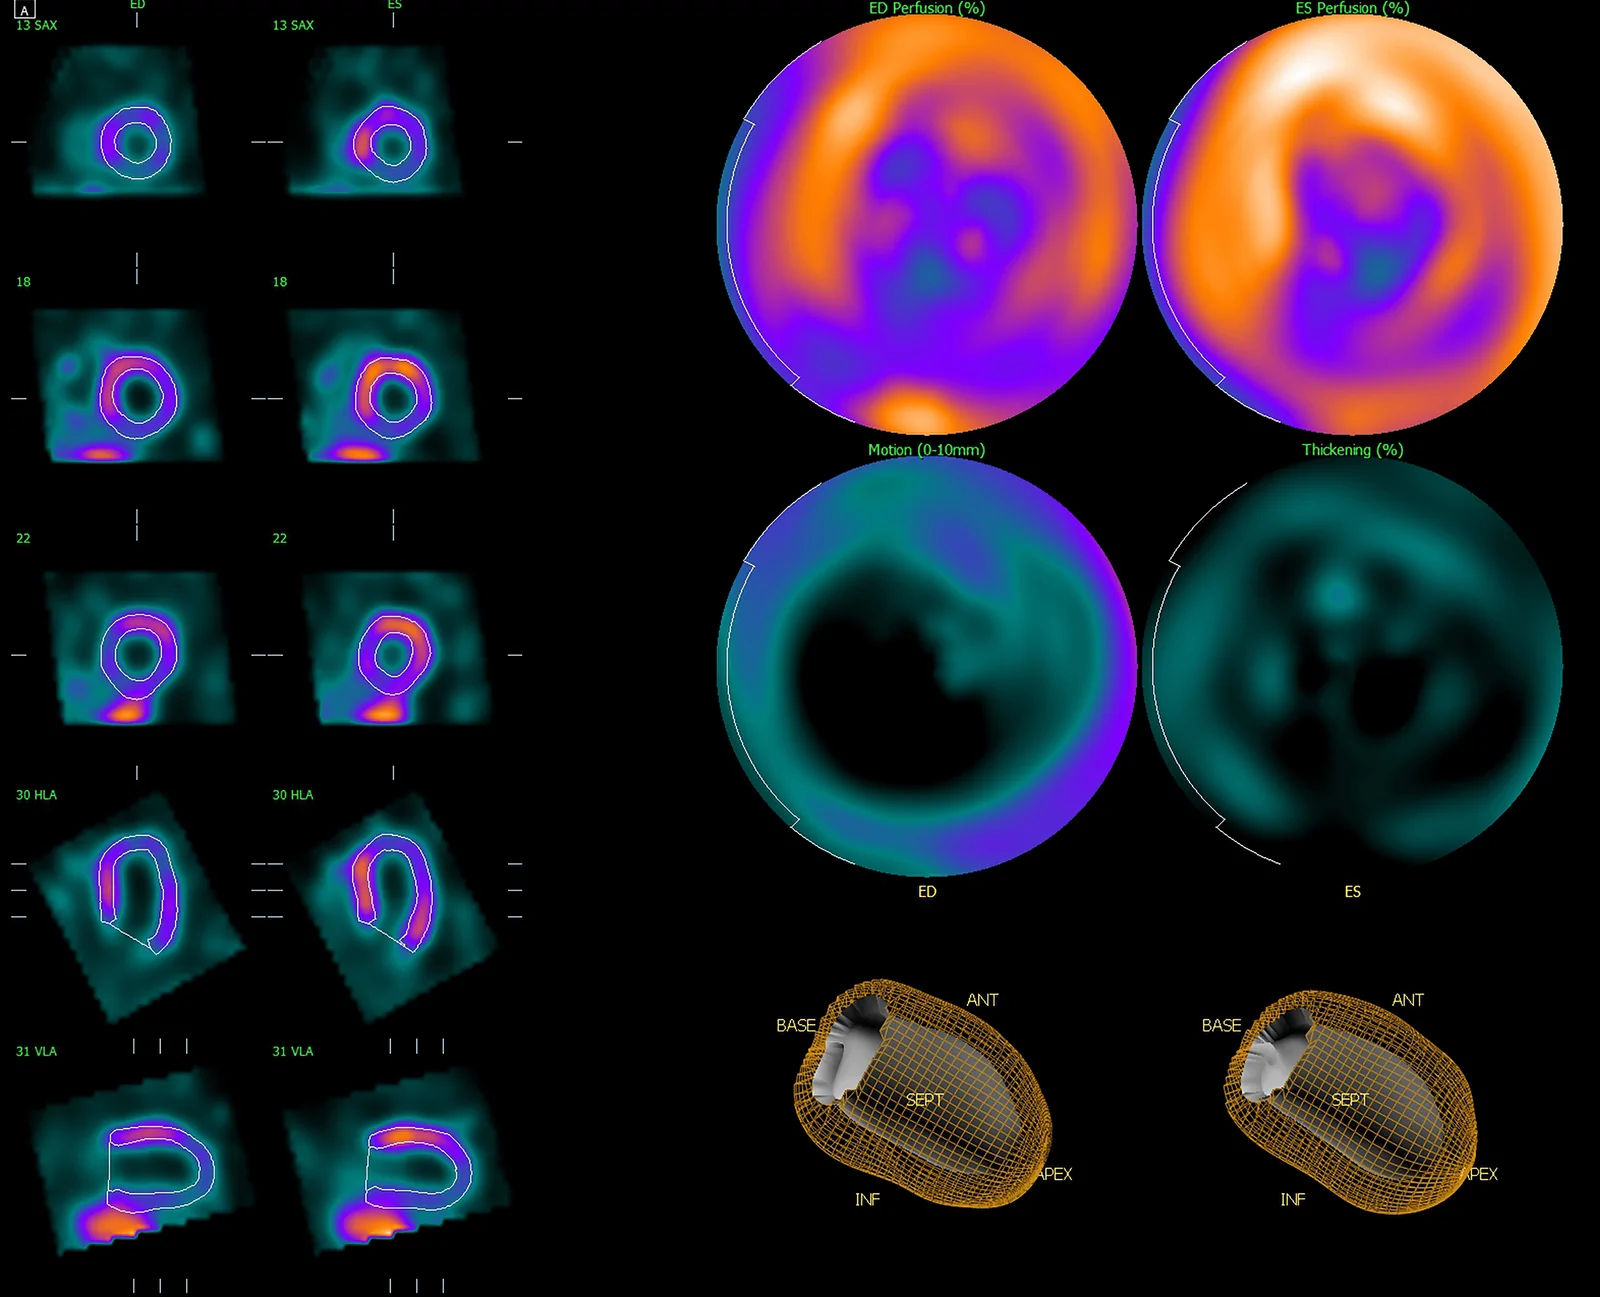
Patchy myocardial tracer uptake abnormalities demonstrated on dynamic single‑photon emission computed tomography (D‑SPECT) myocardial perfusion imaging D‑SPECT myocardial perfusion imaging showing characteristic patchy tracer uptake abnormalities in the apical and mid‑ventricular segments.
